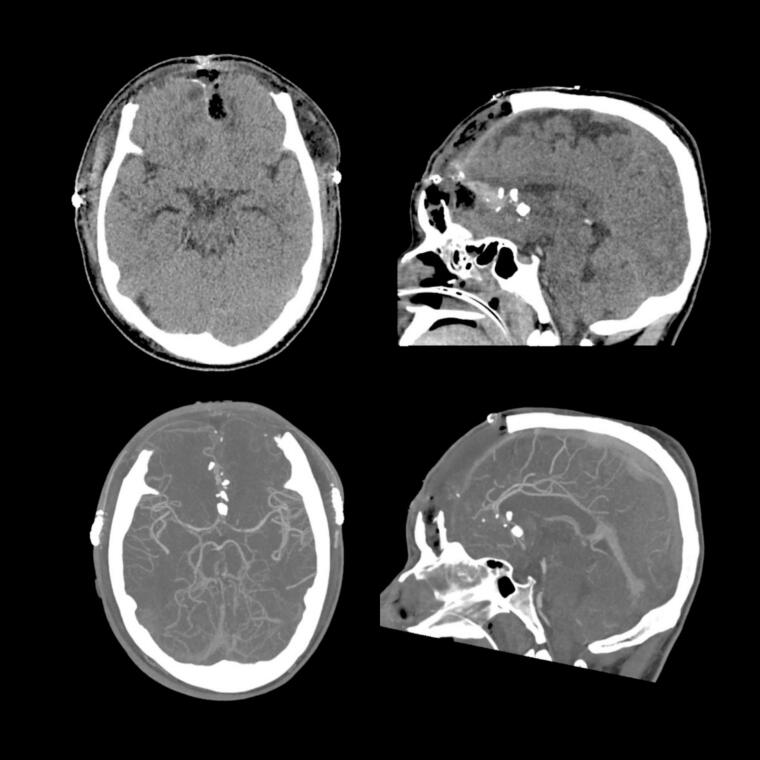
Post operative CT Brain and CT Brain Angiogram (Axial/Sagittal views).
